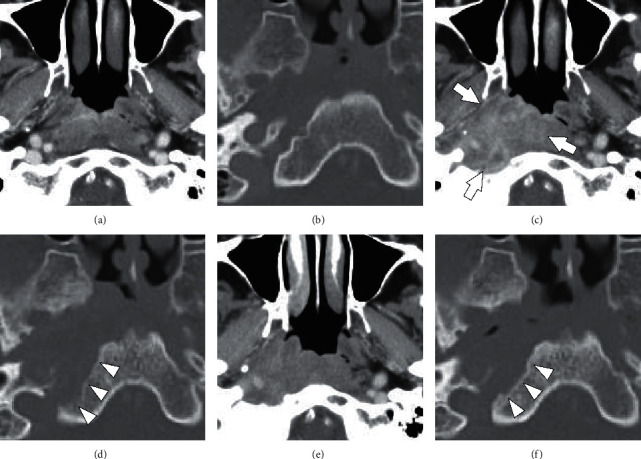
Cervical computed tomography (CT) at the initial visit (a, b) and when glossopharyngeal and vagus nerve paralysis emerged (c, d). CT taken after improvement of symptoms (e, f). The upper panels are contrast-enhanced images of the soft tissue of the nasopharynx (a, c, e). The lower panels are contrast-enhanced images of the bony structure of the extra skull base (b, d, f). The development of diffuse enhancing mucosal irregularity in the nasopharynx (arrow) is shown. (d) The bony erosion of the external skull base (arrow head). (f) The improvement of bony erosion (arrow head).
